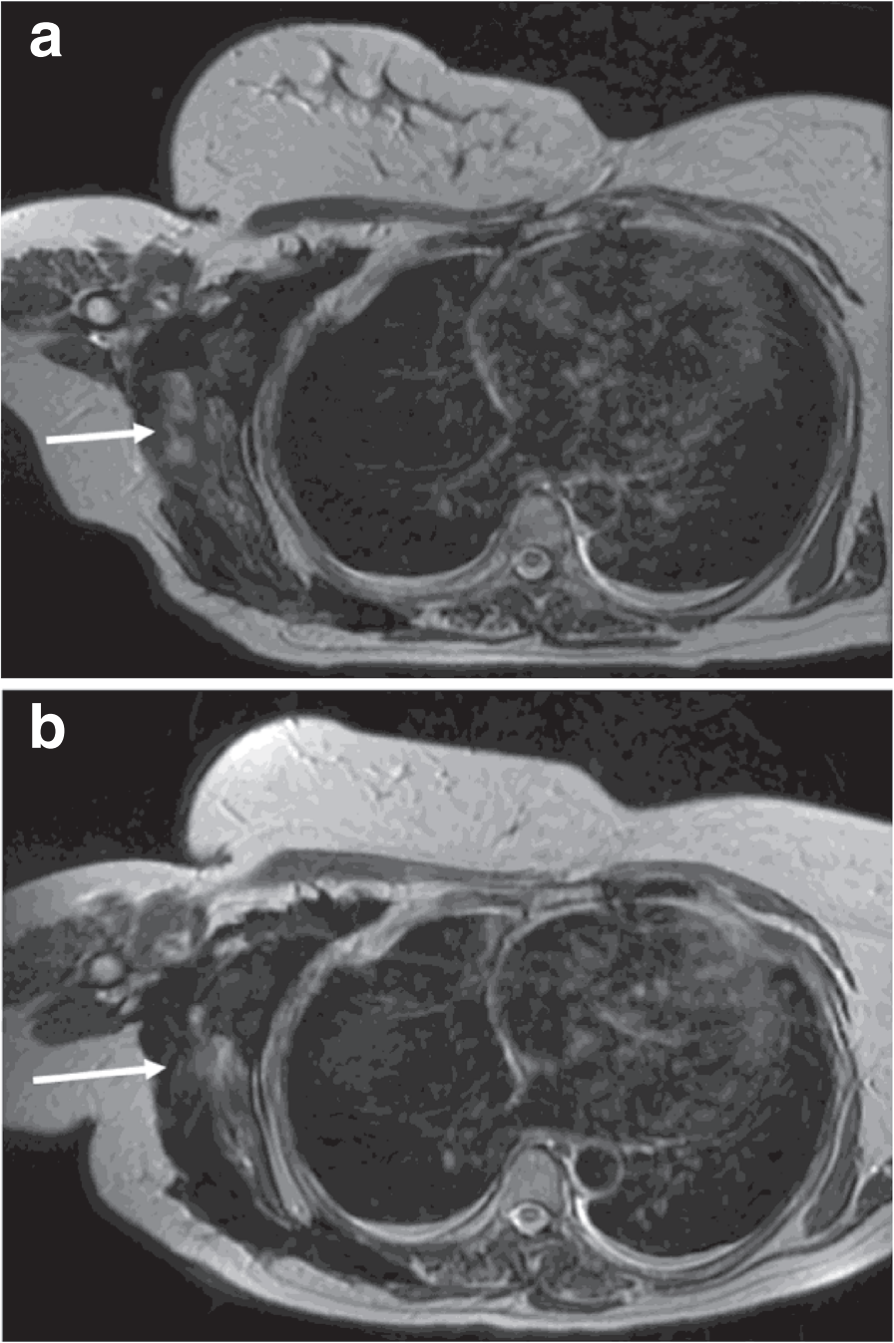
Axial T2 weighted MRI images of the thorax at baseline (a) and following 10 months of pazopanib (b). A large plaque of fibromatosis encases the lateral right hemithorax extending into the axilla. Although the disease remained dimensionally stable the lateral component (arrows) demonstrated T2 signal drop from intermediate to low indicating a reduction in cellularity.
